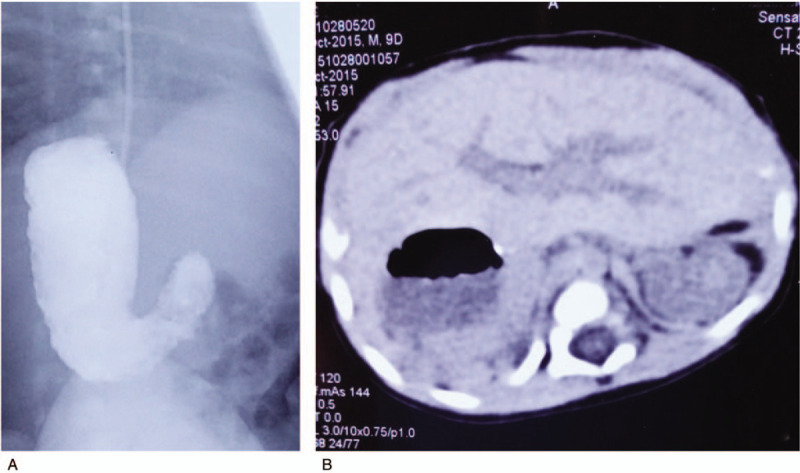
Postoperative 7th day re-examination of the upper gastrointestinal series revealed good contrast echo without extravasation (A). Computed tomography scan indicated situs inversus abdominus (B).
